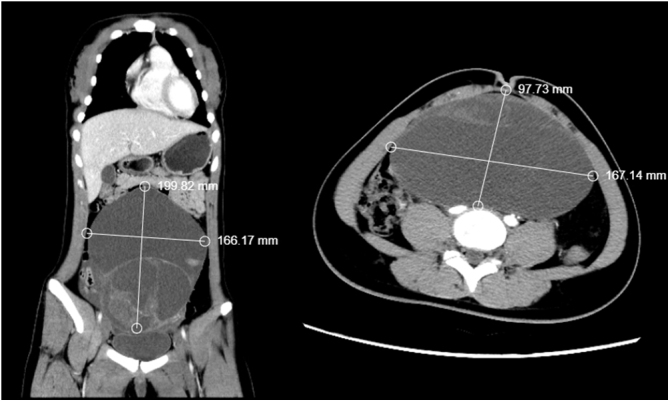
The CT shows a huge cystic-solid mass in the pelvic and abdominal cavity.
